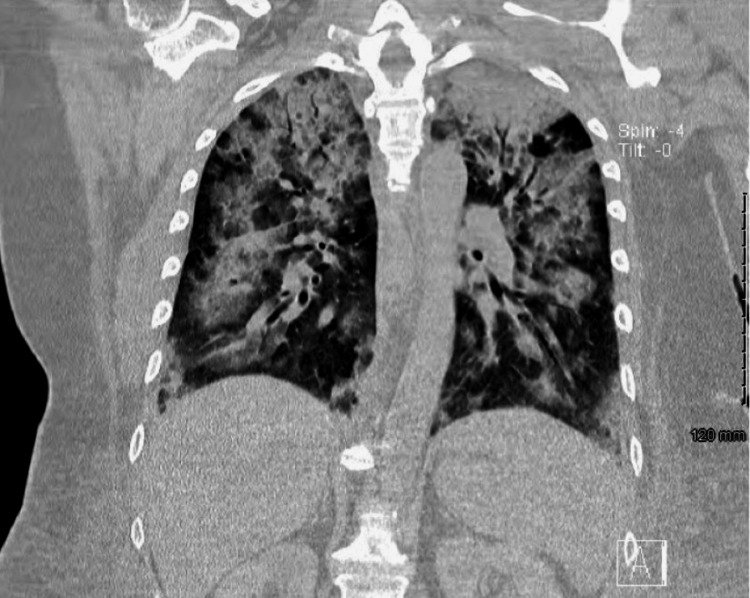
CT chest without contrast The image demonstrates bilateral pleural effusions, patchy mixed ground-glass and consolidative opacities, nonobstructive mixed atelectasis, and consolidation in the lung bases CT: computed tomography
